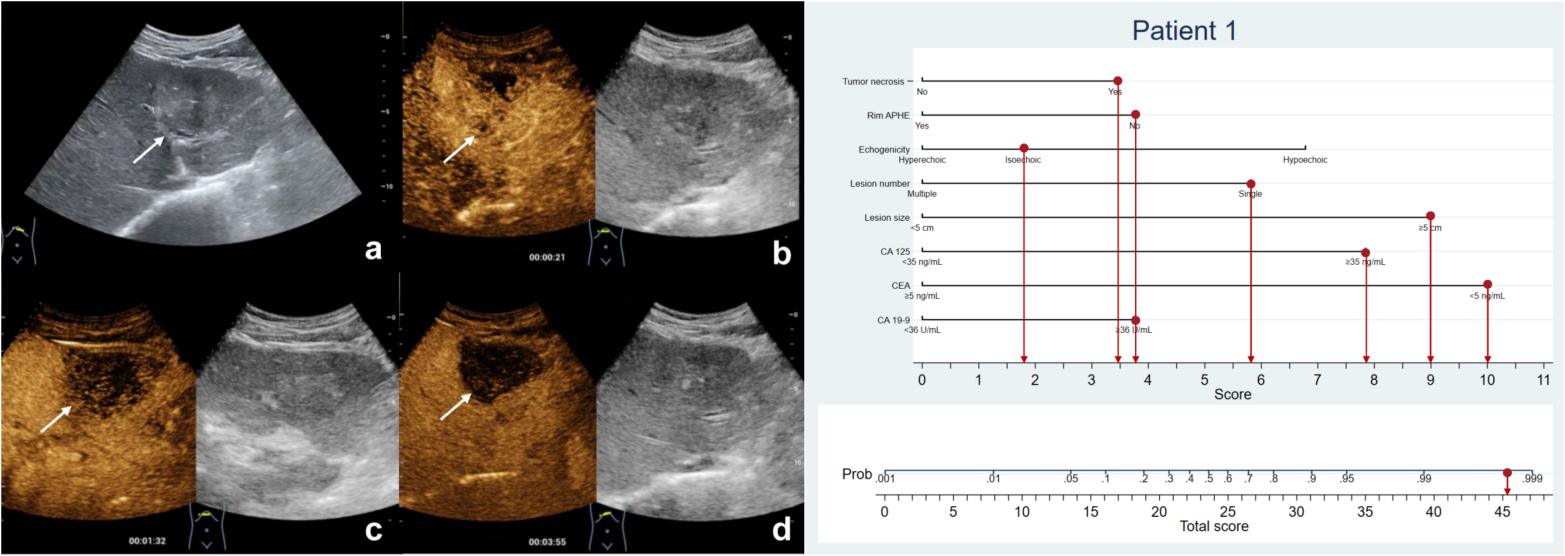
The patient was a 57-year-old man with a 6.1-cm isoechoic solid lesion in the liver (A). Hetero-hyperenhancement was observed during the arterial phase (B), and the lesion showed necrosis areas in the central on contrast-enhanced ultrasound (B–D). The serum level of CA125 and CA19-9 were elevated, while CEA level was normal. Based on the nomogram, a total of 45.2 points were assigned to the patient, corresponding to a probability of more than 90% of having MF-ICC. Postoperative pathological examination confirmed the diagnosis of MF-ICC.
